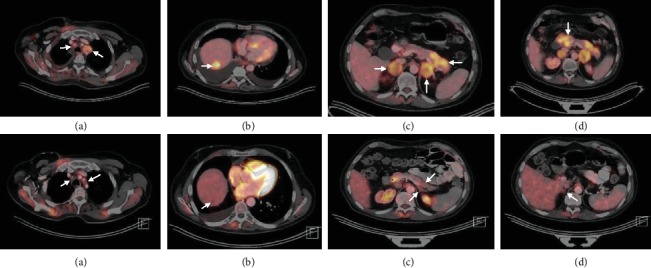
Top—PET-CT chest, abdomen, and pelvis before initiation of nivolumab (July 2015) showing R para-tracheal soft tissue thickening and 3.2 cm L para-tracheal lymph node (a), 2.6 cm R hepatic dome lesion (b), 3.8 cm R adrenal and 4.1 cm L adrenal nodules (c), and multiple pancreatic lesions in the head, body, and tail (c, d). In addition (not shown), the patient had other metastases involving bones, mediastinal and retroperitoneal lymph nodes, and pleural and peritoneal surfaces. The maximum SUV values for R-para-tracheal thickening, L para-tracheal lymph node, hepatic lesion, R adrenal nodule, L adrenal nodule, and pancreatic lesions were 5.2, 6.83, 13.08, 11.9, 9.9, and 11.4, respectively. Bottom—PET-CT chest, abdomen, and pelvis after 16 weeks of nivolumab (December 2015) showing resolution of L para-tracheal lymphadenopathy (a), decrease in R hepatic dome lesion to 1.2 cm (b), decrease in L adrenal nodule to 1.4 cm (c), and resolution of R adrenal (d), and all pancreatic lesions (c, d). Other metastatic lesions (not shown) in the bone, mediastinal and retroperitoneal lymph nodes, and pleural and peritoneal surfaces have completely resolved. The maximum SUV for R hepatic dome lesion has reduced from 13.08 to 2.0, and no increased metabolic activity was detected in the L adrenal nodule. Other than equivocal increased metabolic activity in previously irradiated R para-tracheal region (SUV 2.4), there was no increase in metabolic activity anywhere else.
